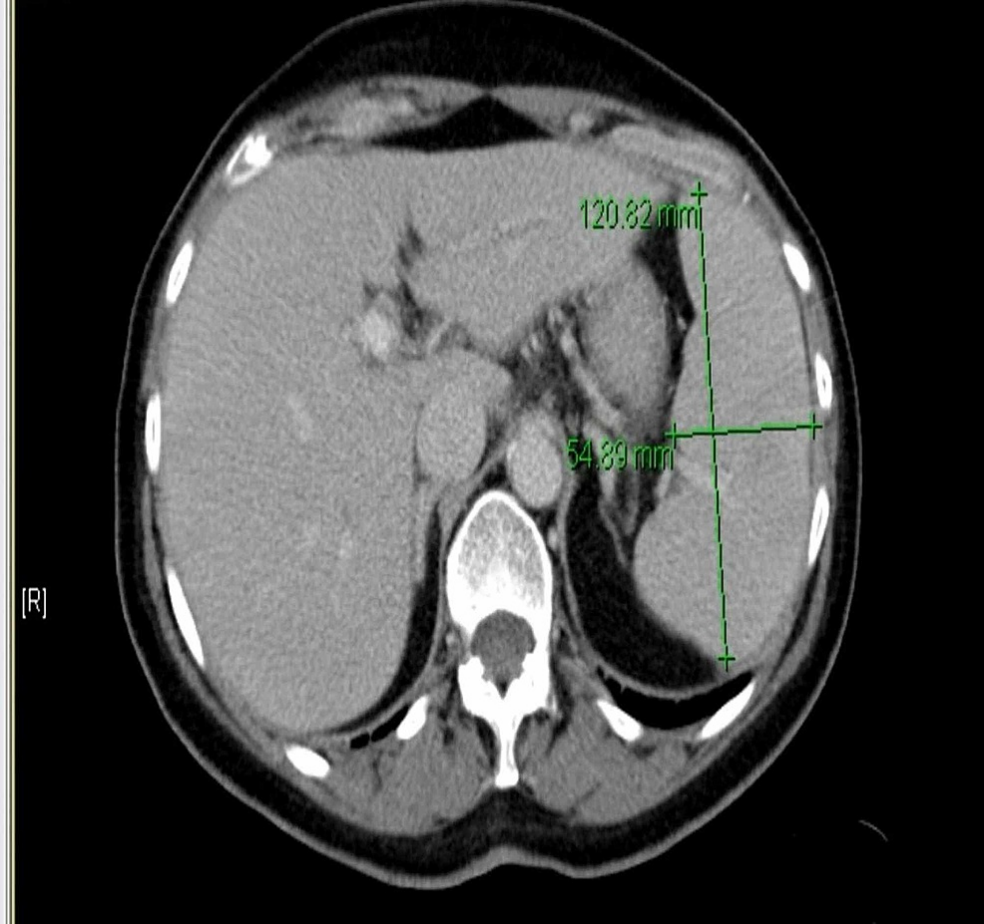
Cross section of the abdomen/pelvis CT scan with contrast showing splenomegaly (spleen measurement shown with green lines)
